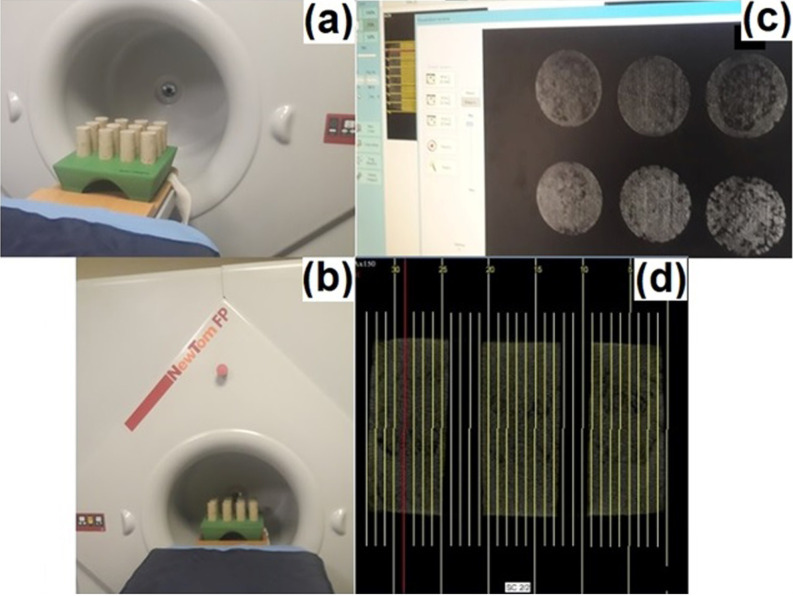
CT imaging unit (a- CT cabinet b- Sample tray, c- Cross section top views of slice samples, d- Longitudinal section of samples).
